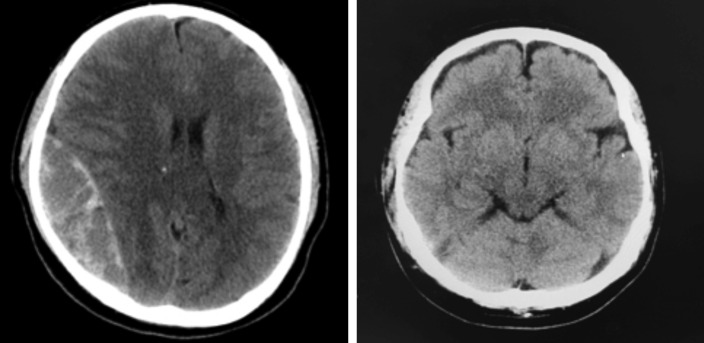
CT brain; (a) Right sided intracranial subdural hematoma at post-operative day three. (b) Resolution of hematoma after evacuation.
